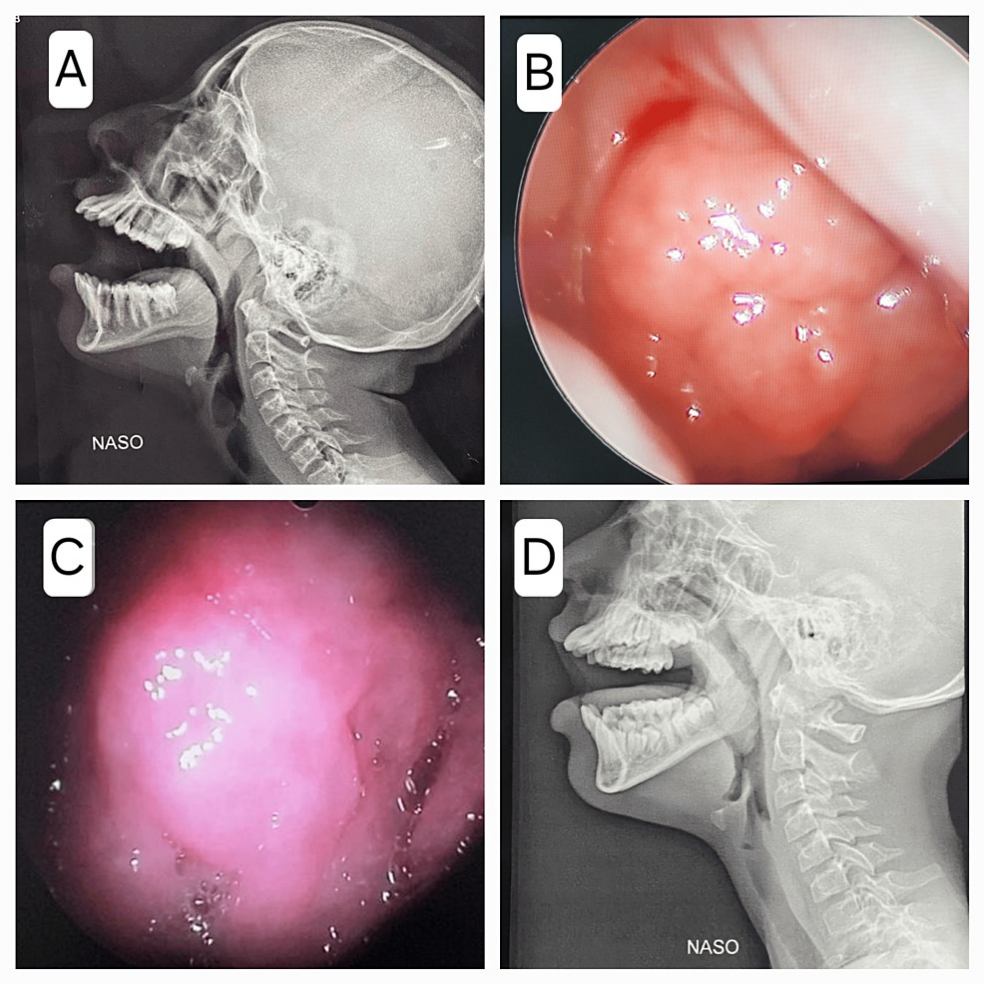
B shows the endoscopic image of the adenoid and A is its corresponding X-ray. C shows the endoscopic image of the adenoid and D is its corresponding X-ray.
